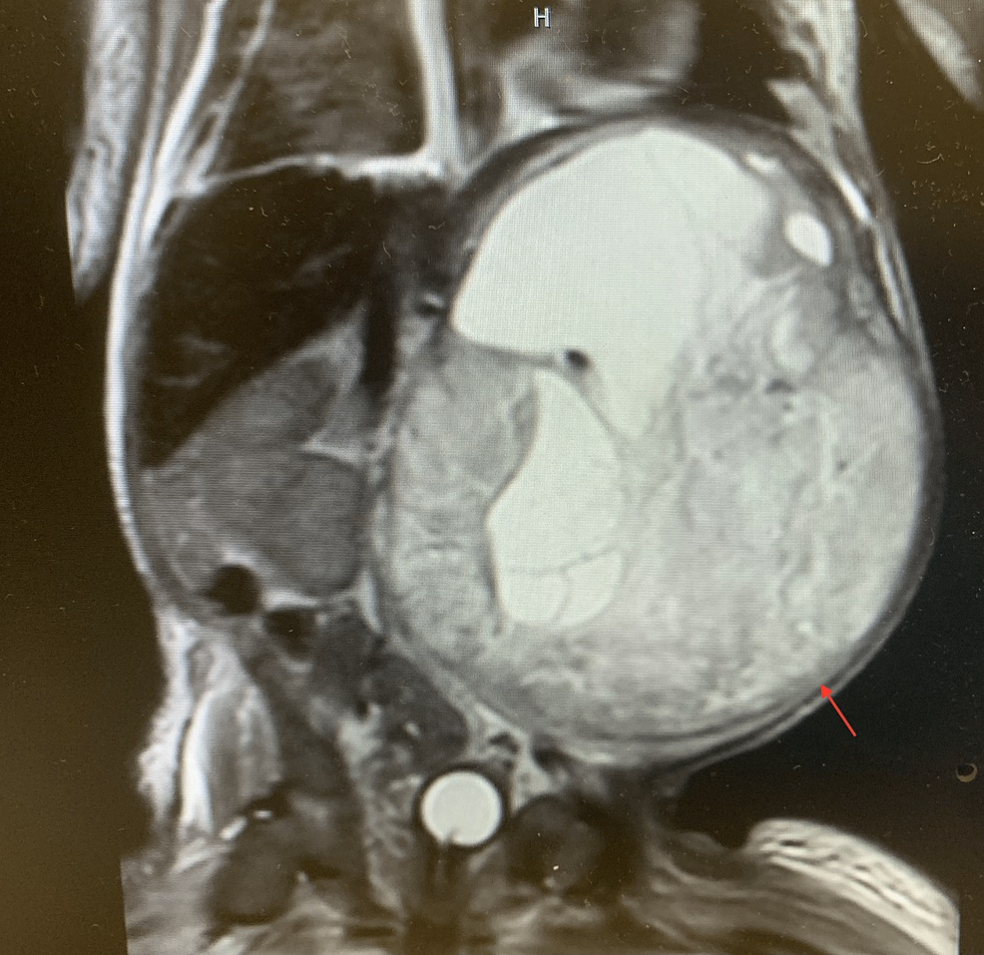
Abdominal MRI coronal image showing tumor burden causing rightward aortic displacement (arrow) MRI: magnetic resonance imaging
